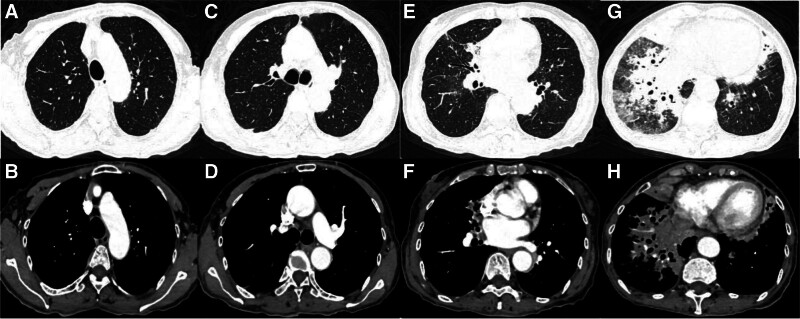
2021-05-30 enhanced scan with thin-section CT: (A, C, E) and (G) were pulmonary windows. The bronchi of the middle lobe of the right lung, the lingual segment of the upper lobe of the left lung, and the lower lobe of both lungs were slightly dilated; the tube wall was thickened; and the surrounding spots were seen, significant in the middle and lower lobes of the right lung; some lung tissue was solid; air bronchi were visible; and vague nodules were seen in both lungs. (B, D, F) and (H) were enhanced mediastinal windows. After enhancement, slight enhancement of solid tissue was observed; calcified nodules were observed under the right pleura; and calcified aorta and coronary wall were observed. CT = computed tomography.
